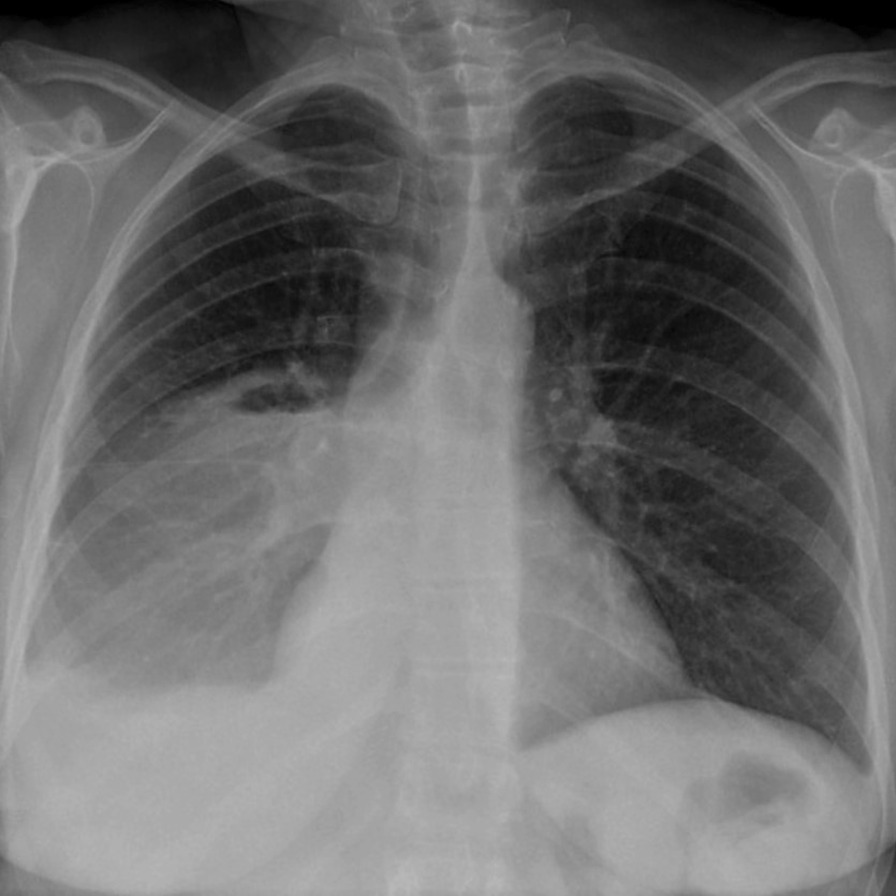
Chest X-ray showing the presence of solid mass peripherally in the lower lobe of the right lung
